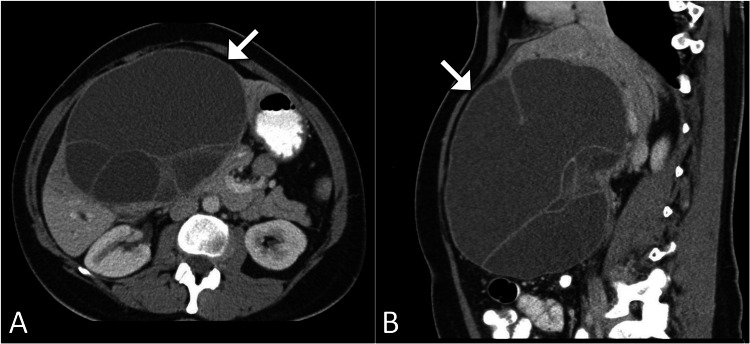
Axial (A) and sagittal (B) CT images depicting a prominent multiloculated hepatic lesion (indicated by arrow) in the right lobe
